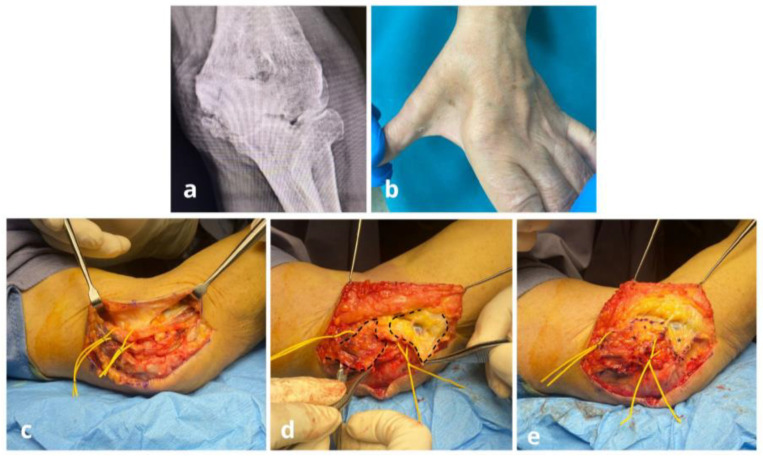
Case n°7. Anteroposterior radiograph of the left elbow in a patient with multiple hereditary exostoses, showing osteophytes in the posteromedial region of the joint (a). Clinically, the patient exhibited severe ulnar nerve impairment, with atrophy of the first web space and claw-hand deformity (b). The ulnar nerve appeared severely compromised. After neurolysis and removal of posteromedial osteophytes, the nerve was anteriorly transposed following excision of the medial intermuscular septum and wide release of the retinaculum and Osborne’s fascia between the two heads of the flexor carpi ulnaris (c). A double freestyle flap was required to provide full-length coverage of the nerve: the proximal portion was covered with a posteromedial freestyle flap supplied by branches of the recurrent ulnar artery, while the distal portion was reconstructed with an anteromedial freestyle flap based on collateral branches of the ulnar artery (d). Final intraoperative view showing definitive ulnar nerve coverage with the double flap (*) (e).
